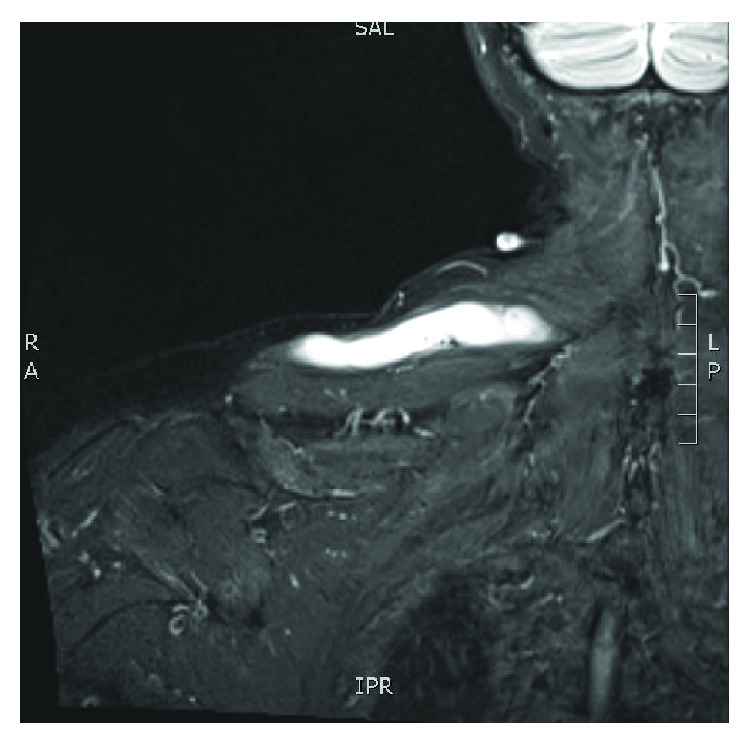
Coronal cuts of MRI of the right thorax depicting cyst measuring 2 cm (AP) × 13 (transverse) × 1.8 cm (craniocaudad).
